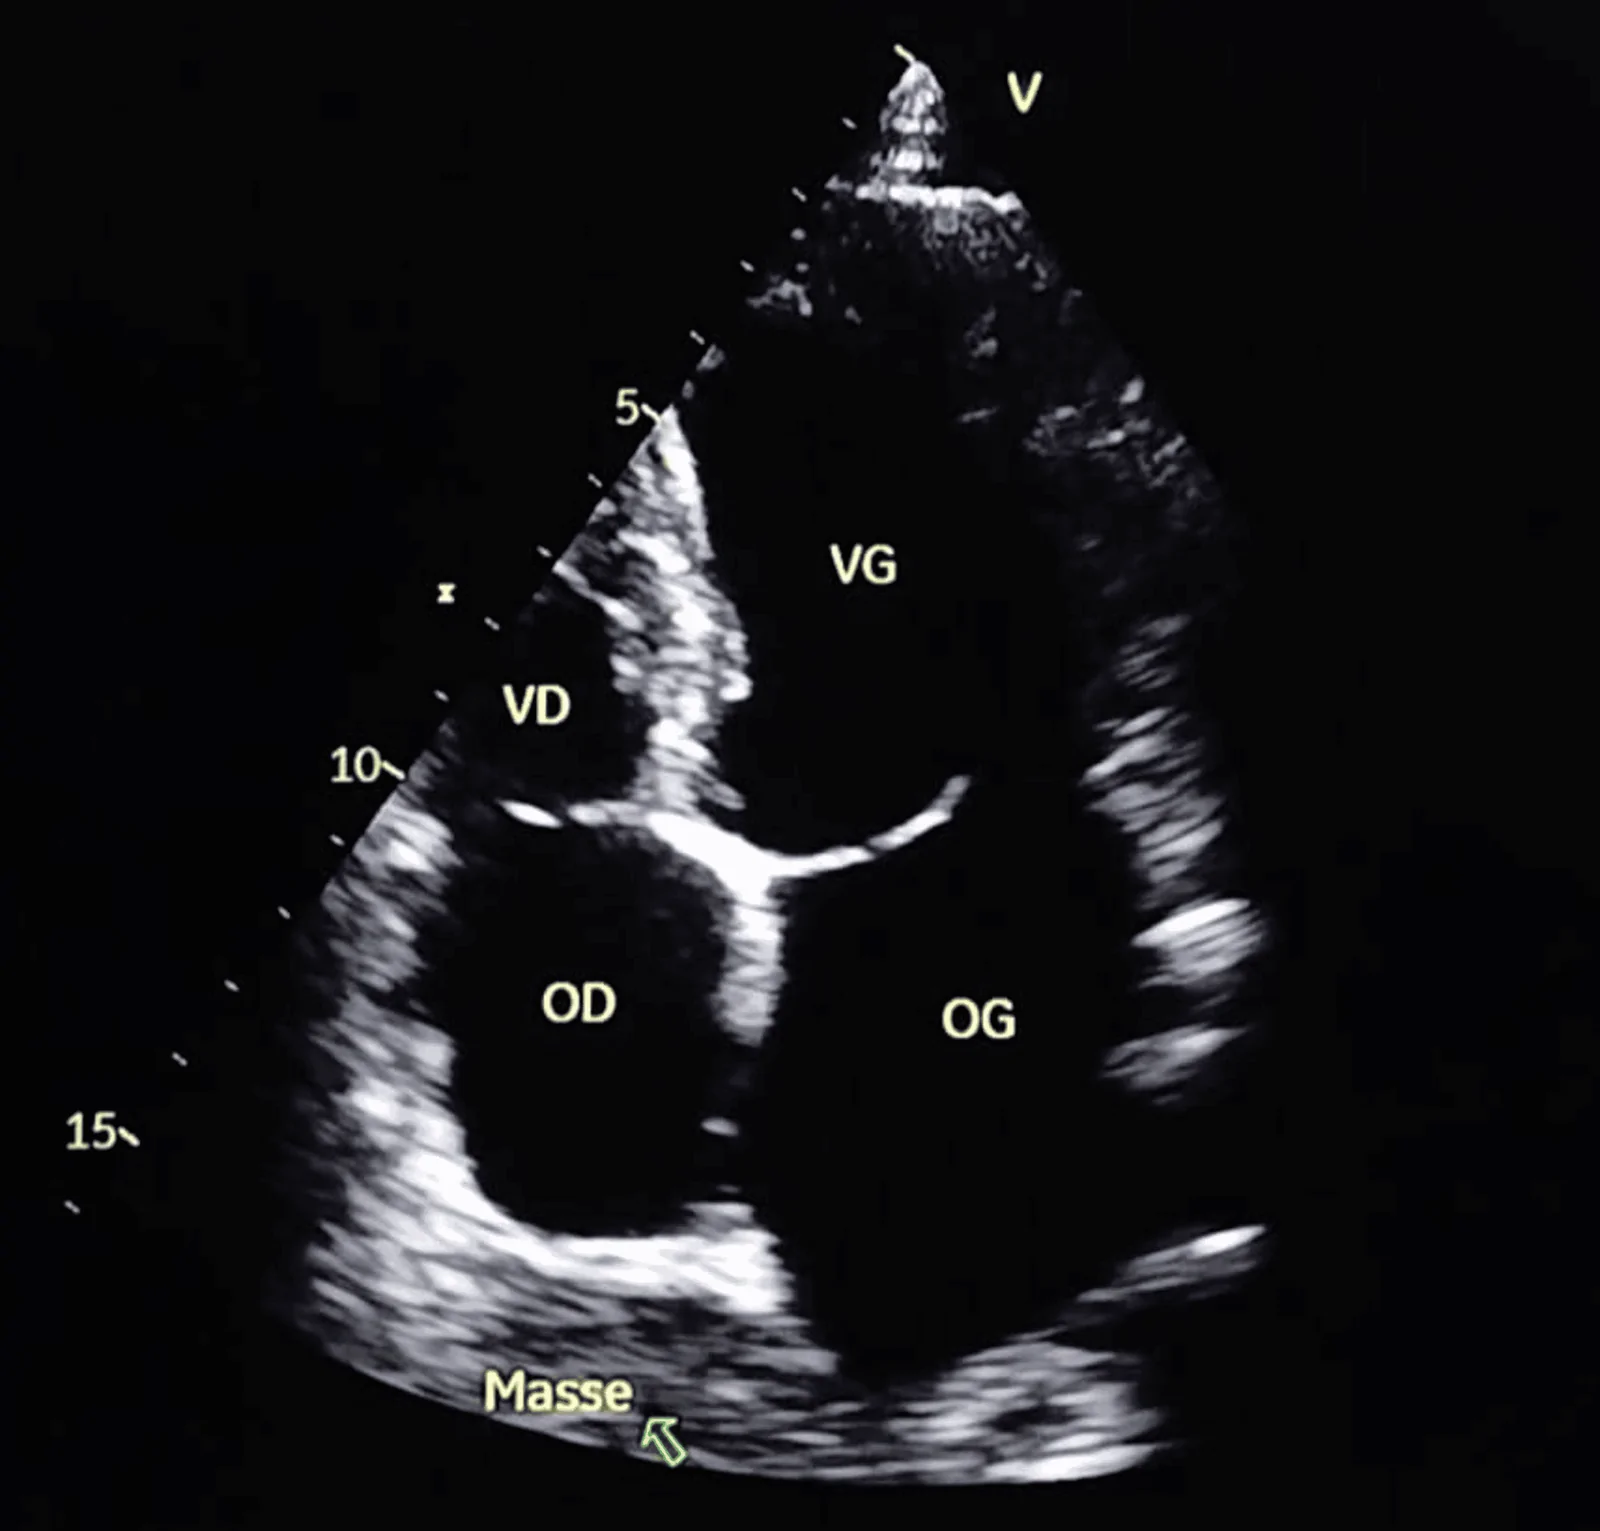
Apical four-chamber echocardiographic view revealing a large mediastinal mass compressing the cardiac structures. OD: *l'oreillette droite* (right atrium); VD: ventricule droit (right ventricle); OG: *oreillette gauche* (left atrium); VG: *ventricule gauche* (left ventricle); Masse: mass (Original labels are in French)
